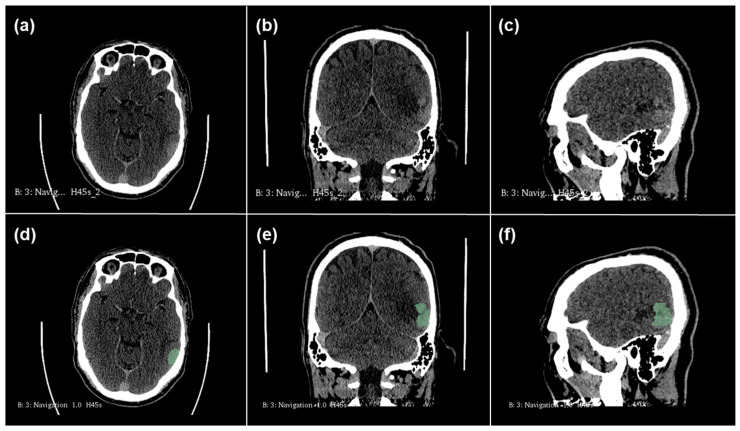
Case of high-grade glioma (HGG). Patient aged 49 years old with astrocytoma localized in left parietal lobe. Images (a–c): original CT images before segmentation. Images (d–f): semi-automatic segmentation with 3D Slicer (areas marked in green).
